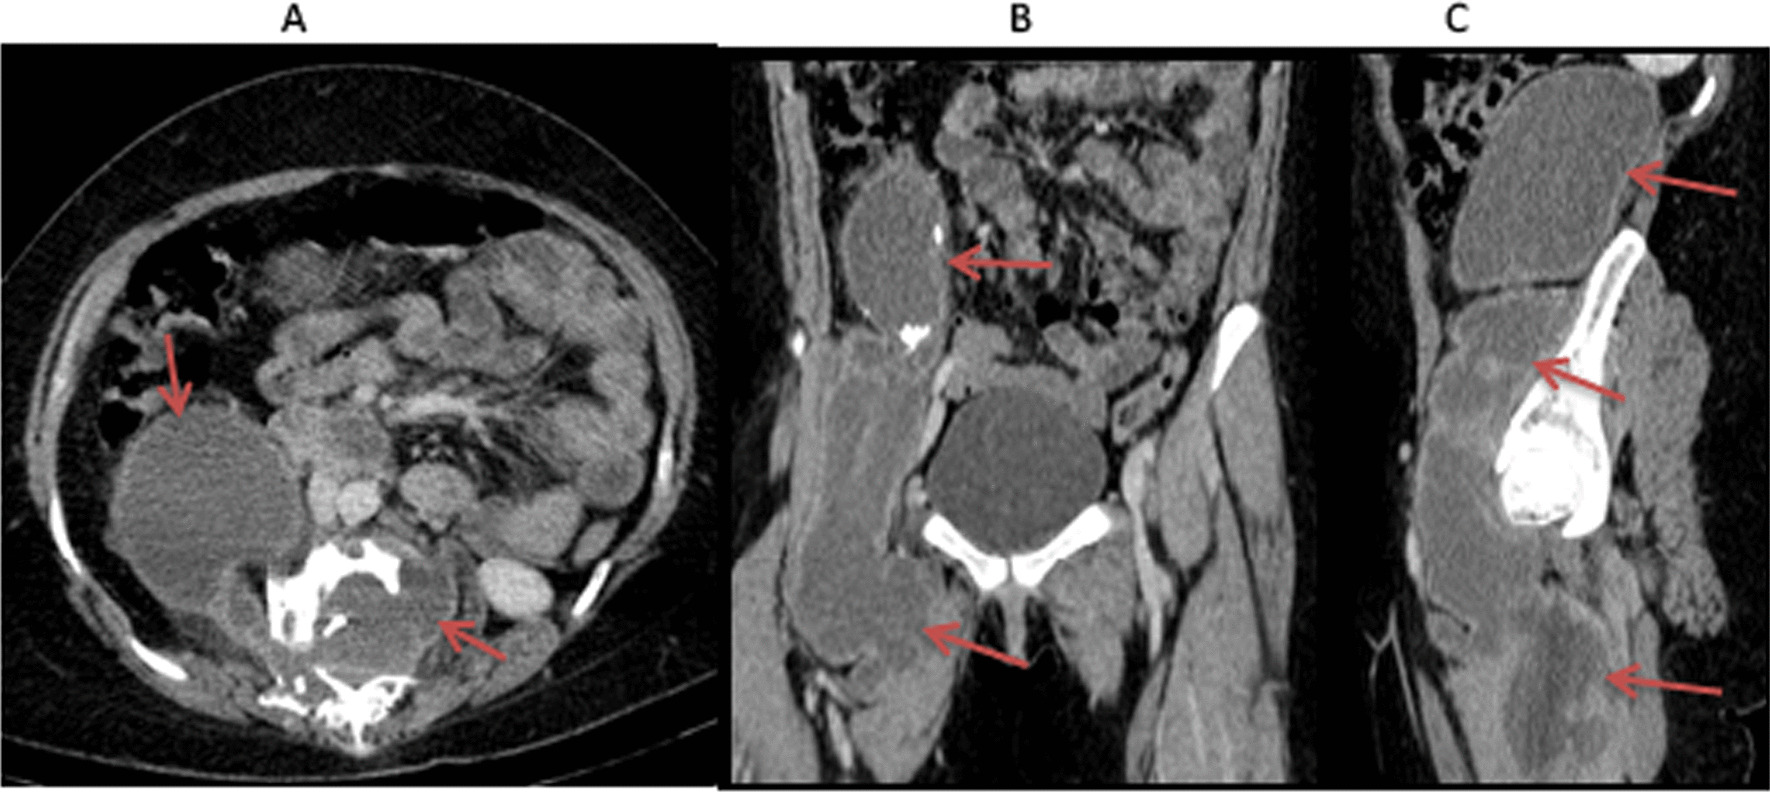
Contrast-enhanced abdominopelvic CT scan: axial (A), coronal (B) and sagittal (C) images demonstrate an extensive paravertebral cystic mass with wall enhancement and calcifications consistent with an abscess (extent of abscess shown by arrows). The abscess extends into the subdural space, and tracks along the retroperitoneal space into the gluteal compartments of the right thigh (best demonstrated on coronal and sagittal images)
